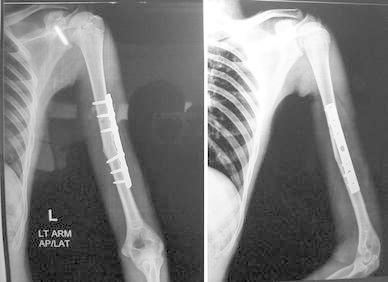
AP and lateral radiographs of the fracture in Fig. 1 shows solid union with DCP after 9 months
